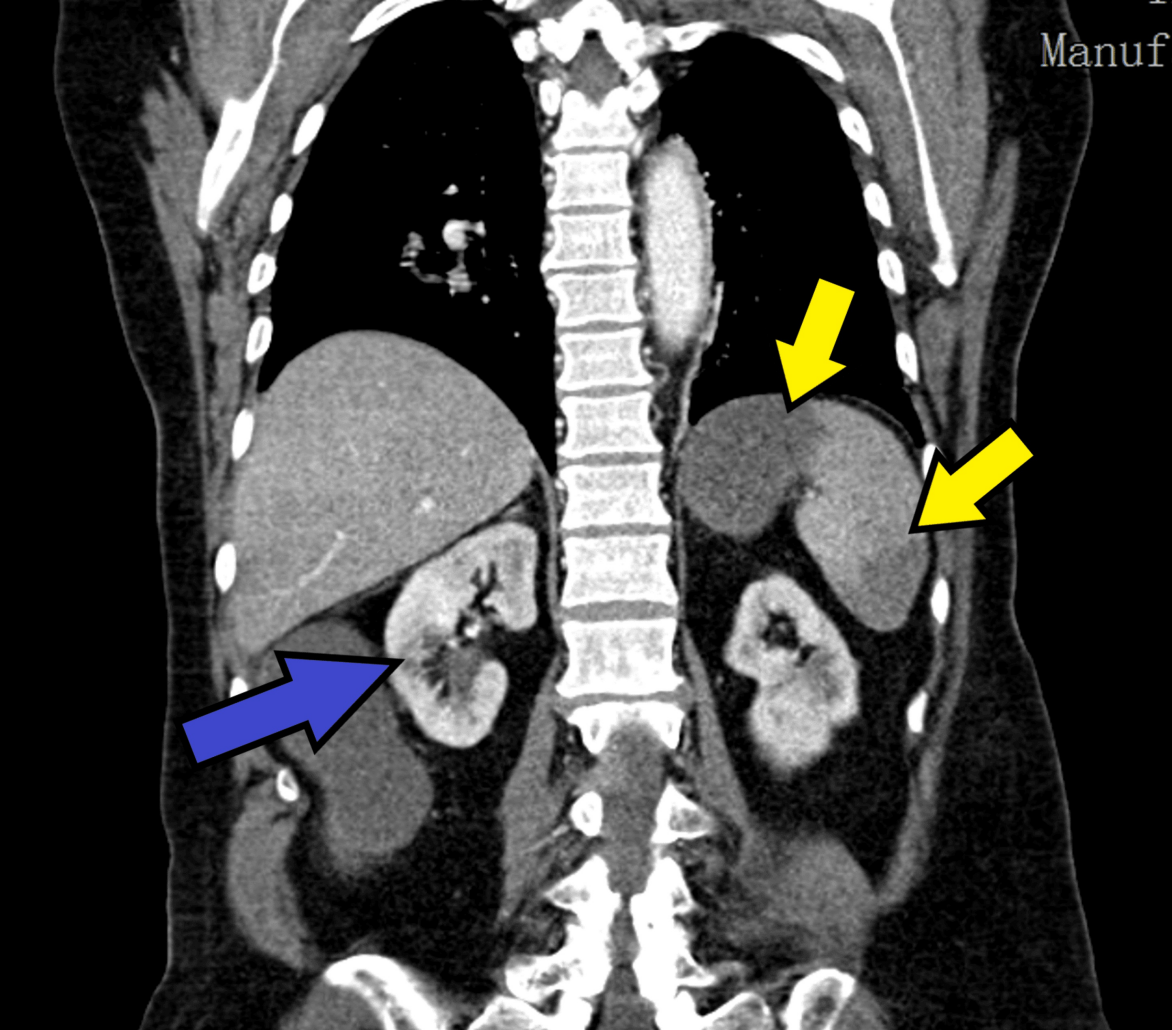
Contrast-enhanced abdominopelvic CT scan Yellow arrow: Splenic infarcts; Blue arrow: Renal infarcts
